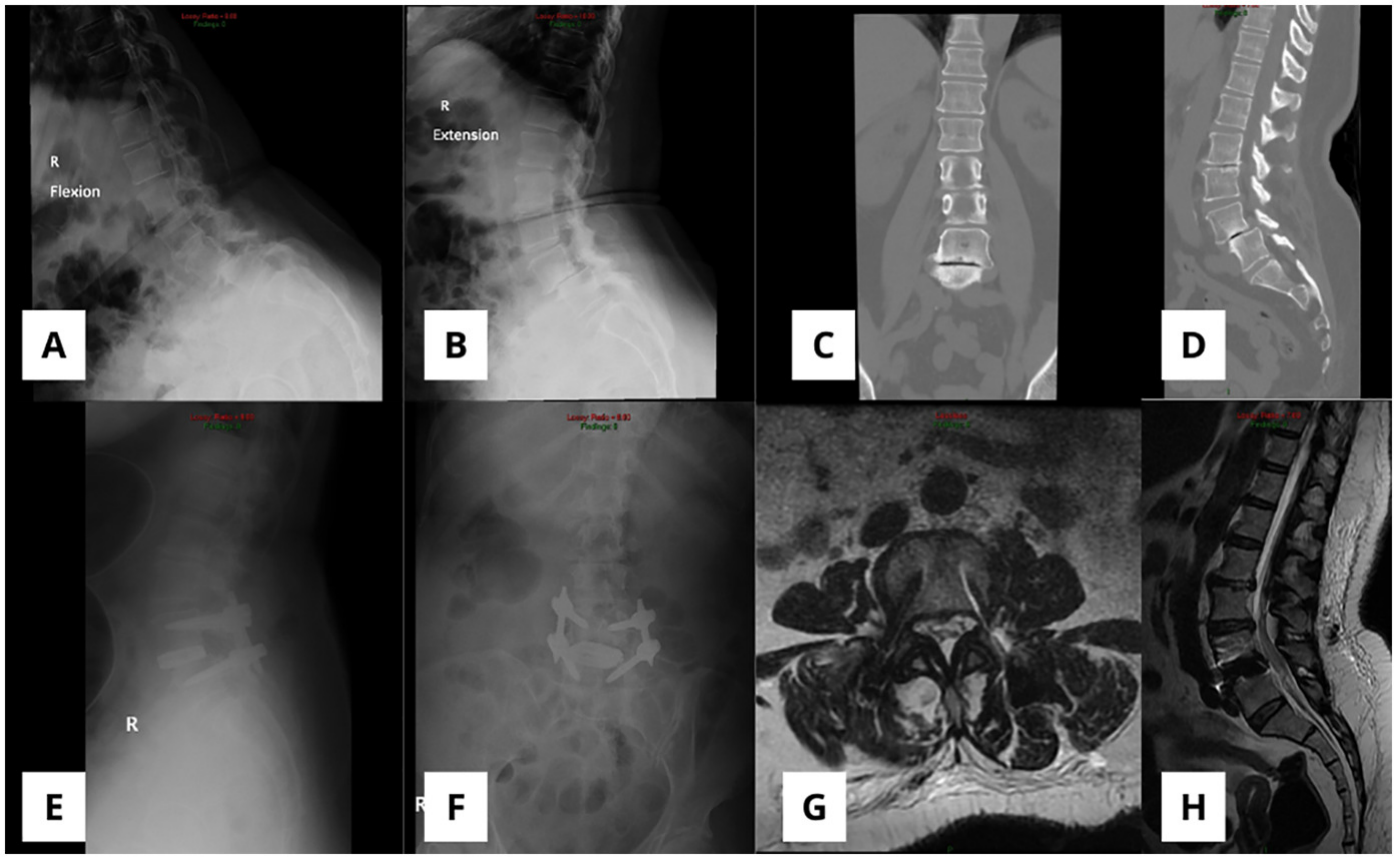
(A,B) X-ray image in lateral flexion and extension showing spondylolisthesis L4/5 before surgery. (C,D) CT scan showed disc narrowing at the level of L5/S1. Postoperative x-ray (E,F) and MRI (G,H) showed that the intervertebral cage was well positioned without displacement.
